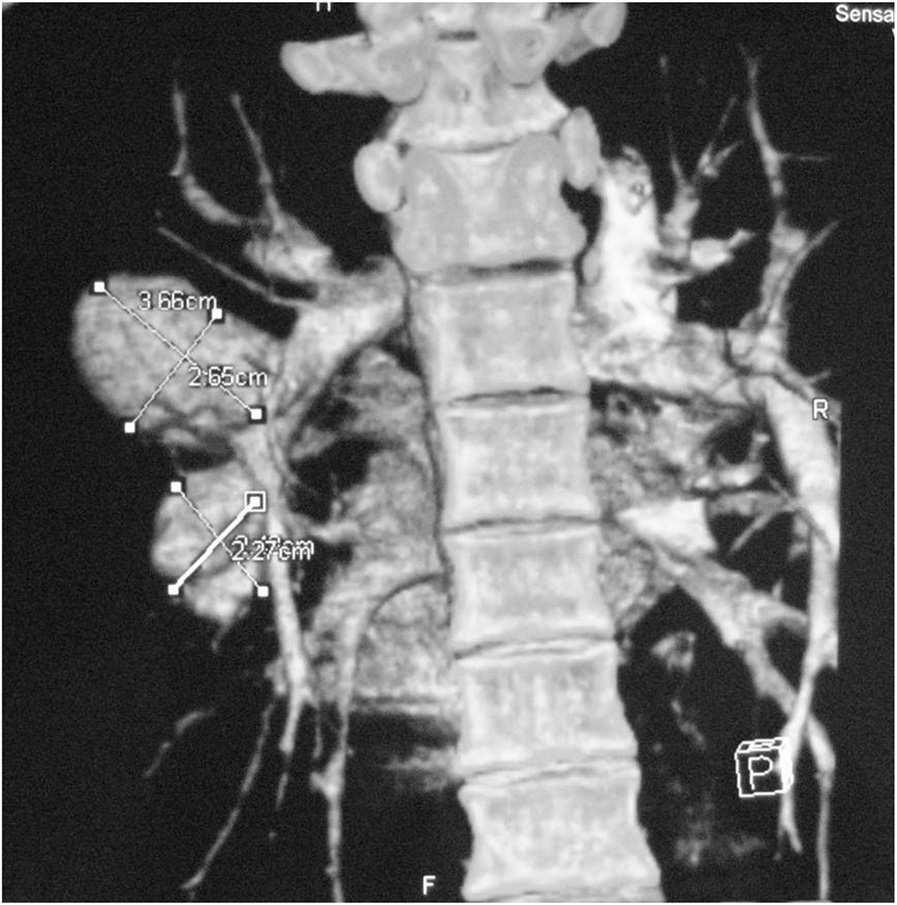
The thoracic MRI is showing the well-shaped, round hilar opacities on left hemithorax.
